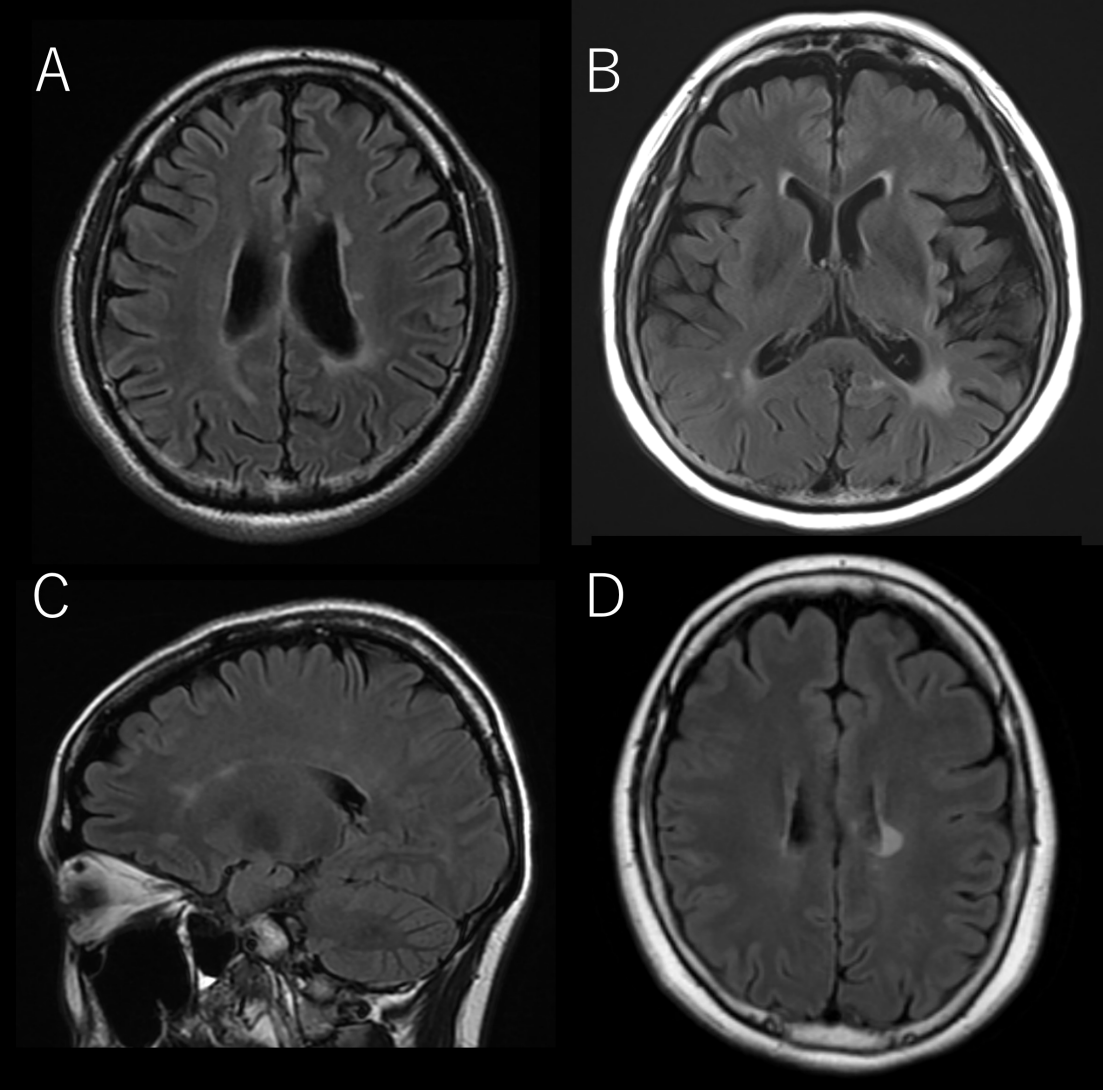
The representative brain MRI of cases that corresponded to the Red Flags indicated in Table 4. (A) OCB-negative-13, (B) OCB-positive-8, (C) OCB-positive-9, (D) OCB-positive-10.
